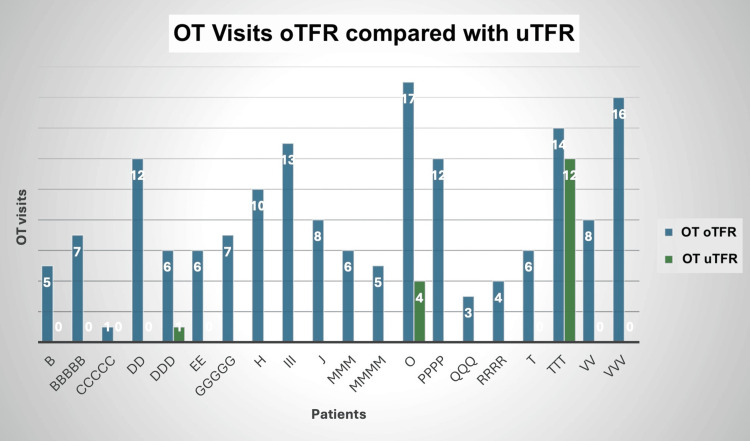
Comparison of OT visits after oTFR or uTFR. The y-axis represents the deidentification codes for individual patients. OT: occupational therapy; oTFR: open trigger finger release; uTFR: ultrasound trigger finger release
